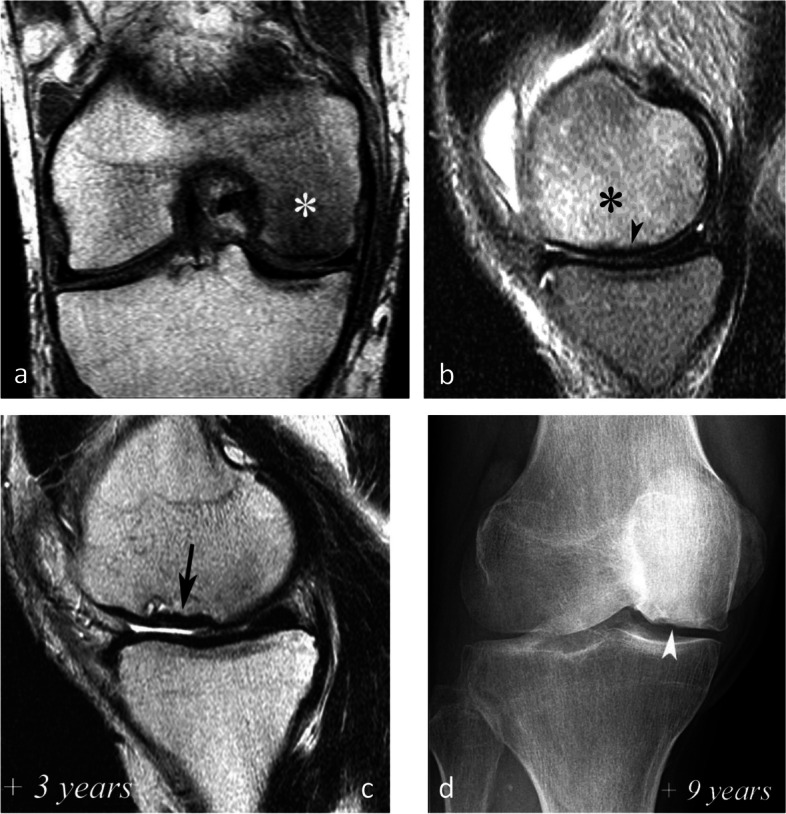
Evolution in two stages of subchondral insufficiency fracture (SIF). a, b Coronal T1-weighted (a) and sagittal T2-weighted (b) MR images show BME-like signal intensities in medial condyle (asterisks), except for a very thin (< 4 mm in thickness) subchondral low signal intensity area on the T2-weighted image (arrowhead in b). c MRI follow-up three years later with T2-weighted image (c) shows a small deformation of the lower pole of the condyle with thickening of the subchondral low signal intensity area, now exceeding 4 mm in thickness (arrows), suggesting the evolution of the SIF towards osteonecrosis (SIF-ON). d Nine years after the initial examination, a radiograph confirms an irreversible lesion, with deformation of the epiphyseal surface (arrowhead), which is moderate in this case and clinically well tolerated
